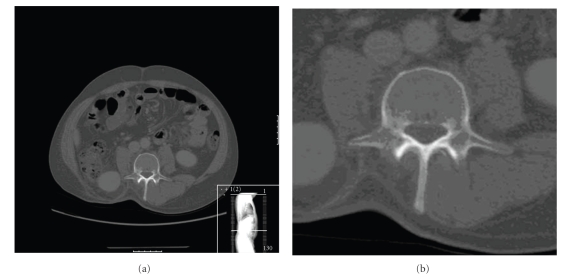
There is a right-sided paraspinal metastatic lesion. The bone windows on CT scan show very subtle lytic change in the vertebral body of L2 which was not considered significant at the time of reporting.
